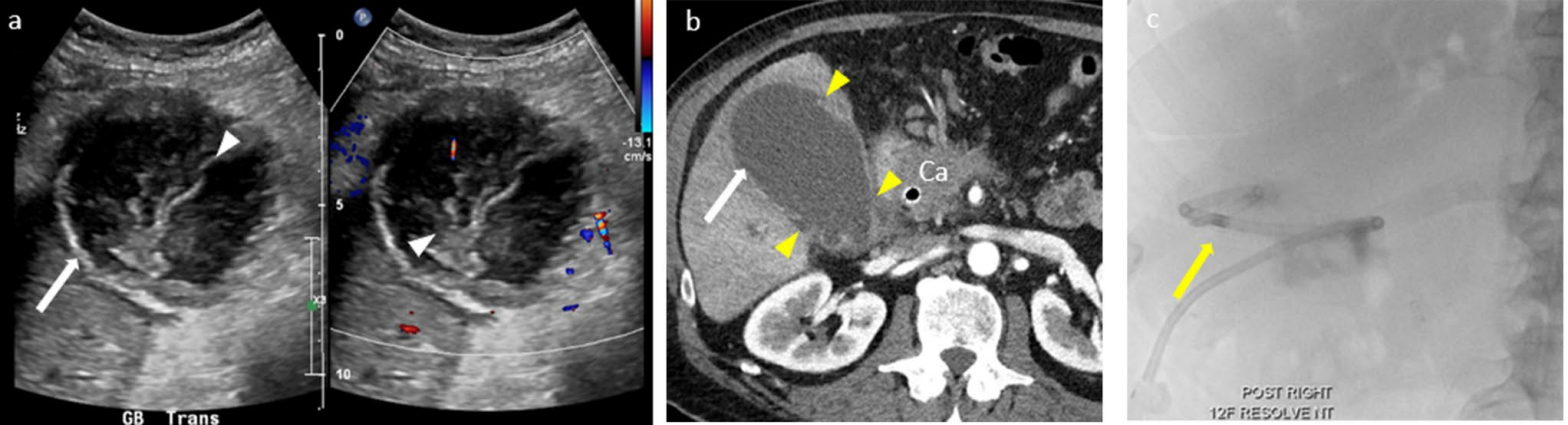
Gangrenous cholecystitis in a 61-year-old male with pancreatic cancer. Ultrasound (image a) demonstrates dilated gallbladder (arrow) with floating linear echogenic intraluminal membranes from sloughed mucosa (arrowheads). Contrast enhanced CT axial image (image b) shows dilated gallbladder (arrow), multiple focal wall irregularities and decreased enhancement due to sloughed mucosa and discontinuity of the wall (yellow arrowheads). A cholecystostomy tube was placed for management (image c, yellow arrow))
